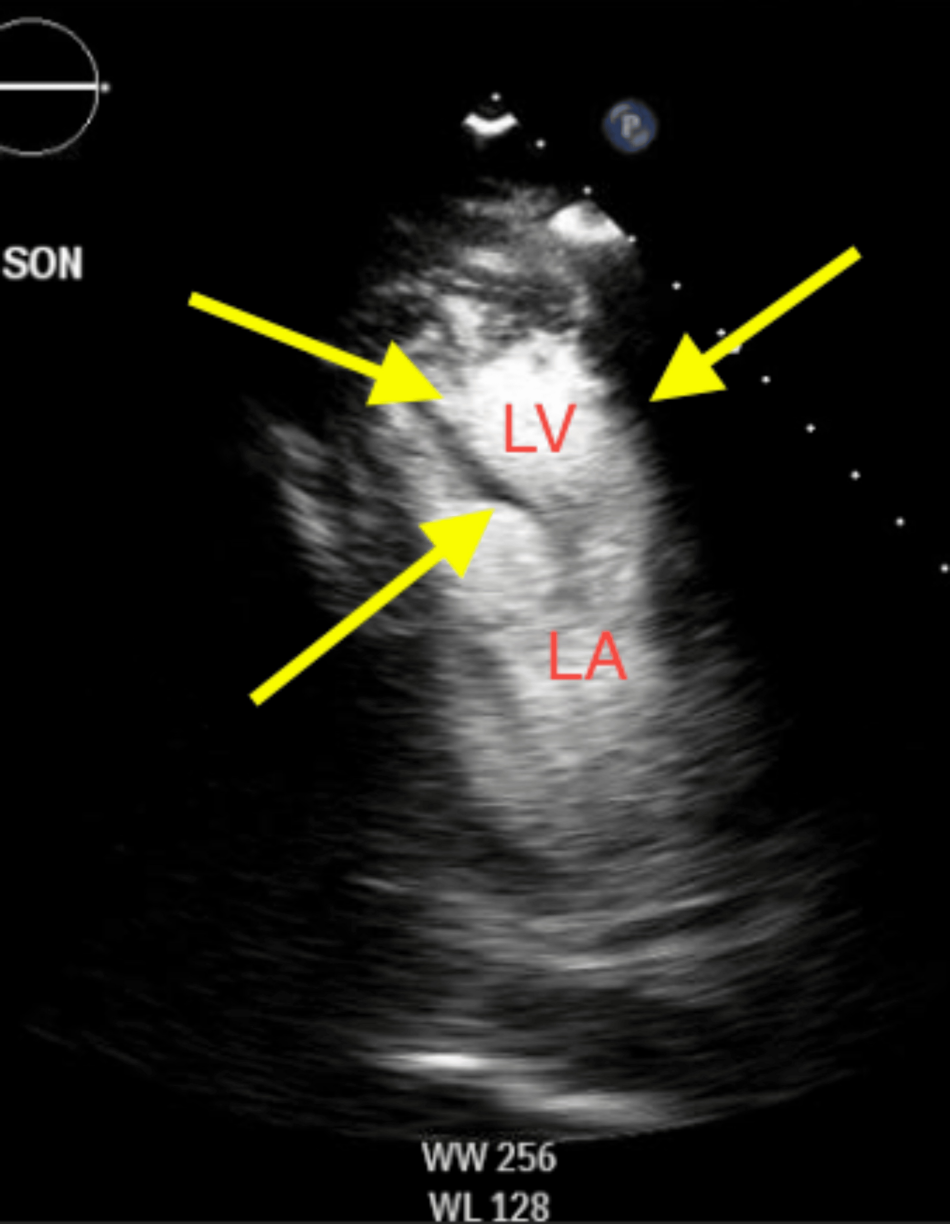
Transthoracic echocardiogram revealing left ventricular apical thrombus (yellow arrows). The two-dimensional apical chamber view depicted thrombus in the left ventricle (yellow arrows). The thrombus is hyperechoic compared to the surrounding myocardial tissue. Right-sided chambers are not well visualized. LV: left ventricle; LA: left atrium.
